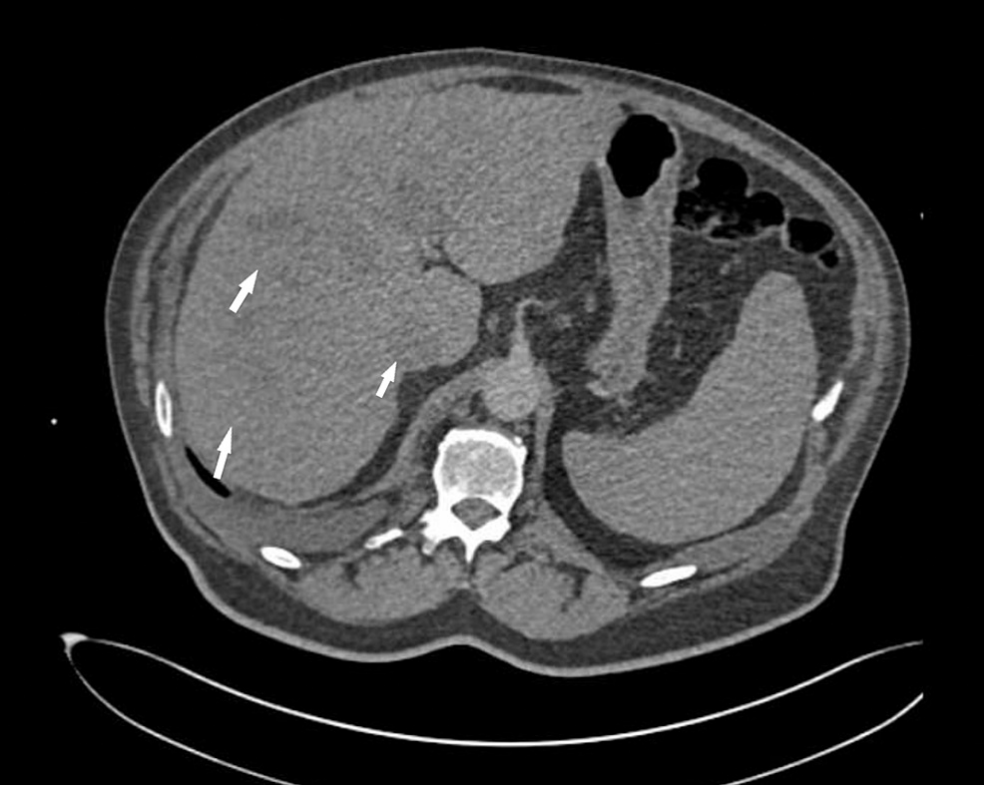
Axial view of CT-Angiogram from presentation showing several metastatic lesions in the liver
